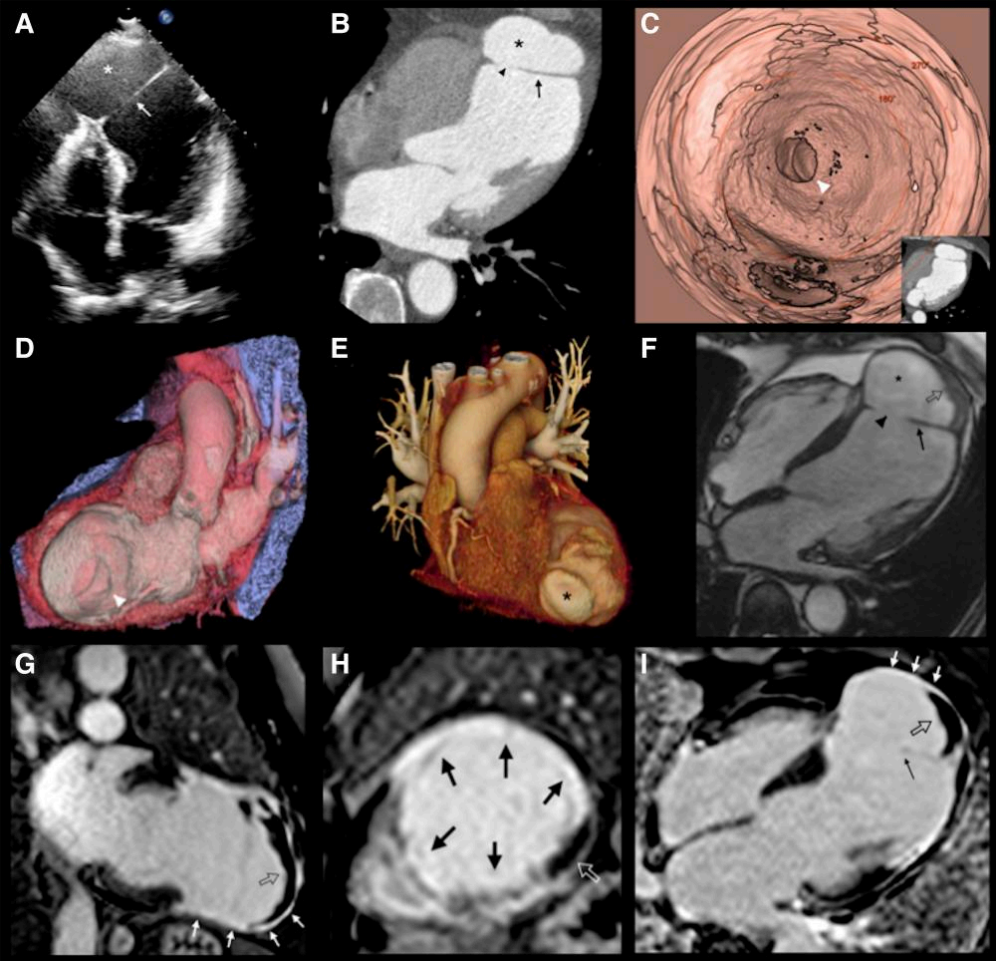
(*A*) Transthoracic echocardiography apical four-chamber (4C) view showing a dissection flap at the apical level (arrow) connecting the left ventricle with a large cavity (*). (*B*) An axial view of cardiac CT confirmed the defect of the myocardial wall (arrowhead) connecting the left ventricle with the cavity (*) previously described by TTE and apparently contained by the pericardium. A false pseudoaneurysm was considered. (*C-D*). Virtual CT ventriculoscopy and long axial 3D VR image with lateral cut showing the defect (arrowhead) in the myocardial wall communicating with the abnormal cavity of the false-pseudoaneurysm. (*E*) Cardiac CT VR reconstruction allowed the visualization of false-pseudoaneurysm from its surface (*). (*F*) CMR apical 4C view with cine showed the presence of thrombus (open arrow) within the false pseudoaneurysm (*), and the communication (arrowhead) through the thinned myocardial wall (arrow). (*G–I*). CMR Phase-Sensitive Inversion Recovery (PSIR) late gadolinium enhancement reconstructions involving the mid-segment of the anterior wall and apical segments (black arrows) and thrombus (open arrow) surrounded by enhancing overlying pericardium (white arrows).
